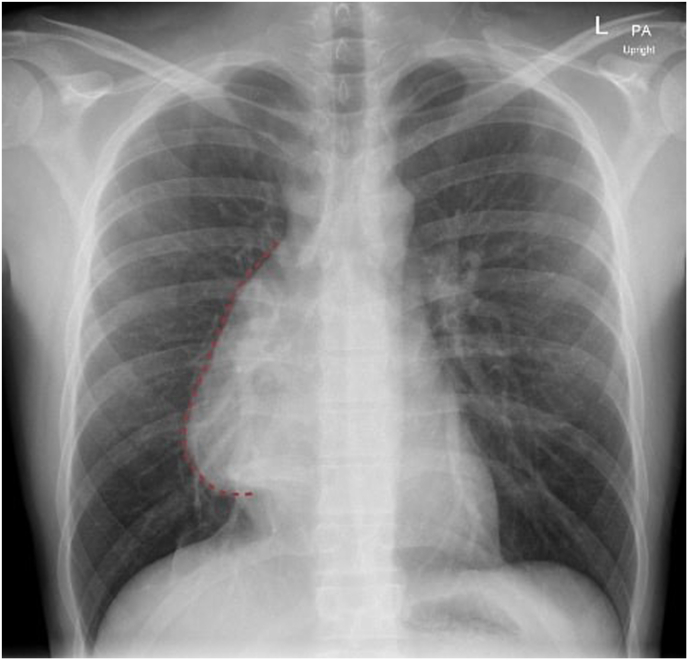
Chest x-ray demonstrating a new prominent density along the right heart border, suspicious of an anterior mediastinal mass.
